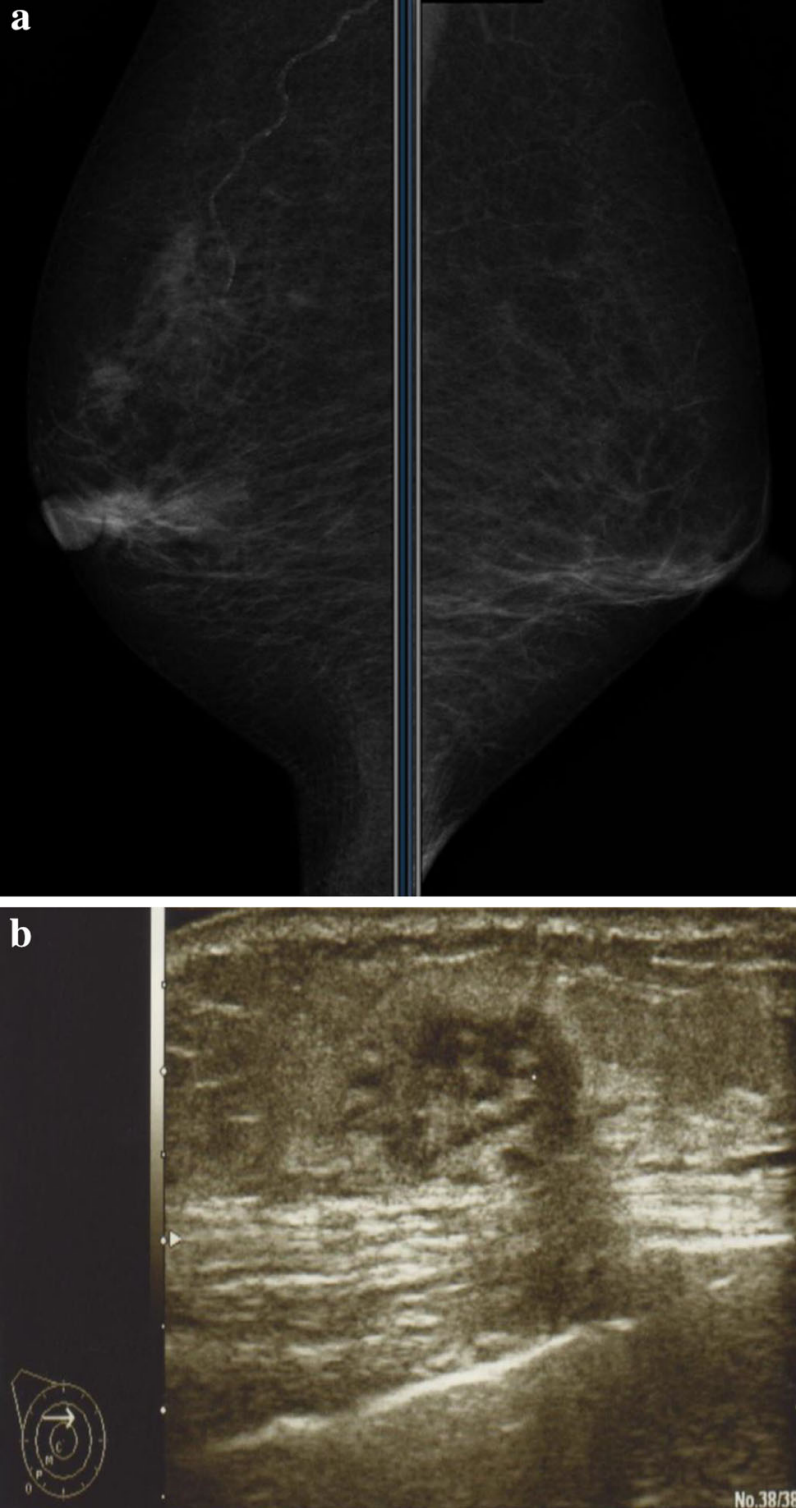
a Mammogram findings. The craniocaudal and mediolateral oblique (MLO) view revealed no mass lesion or other abnormality in the bilateral breasts. b Ultrasonography findings. An irregular, round and solid hypoechoic nodule in the 11:00 o’clock position corresponds to a nodule palpated on clinical examination
